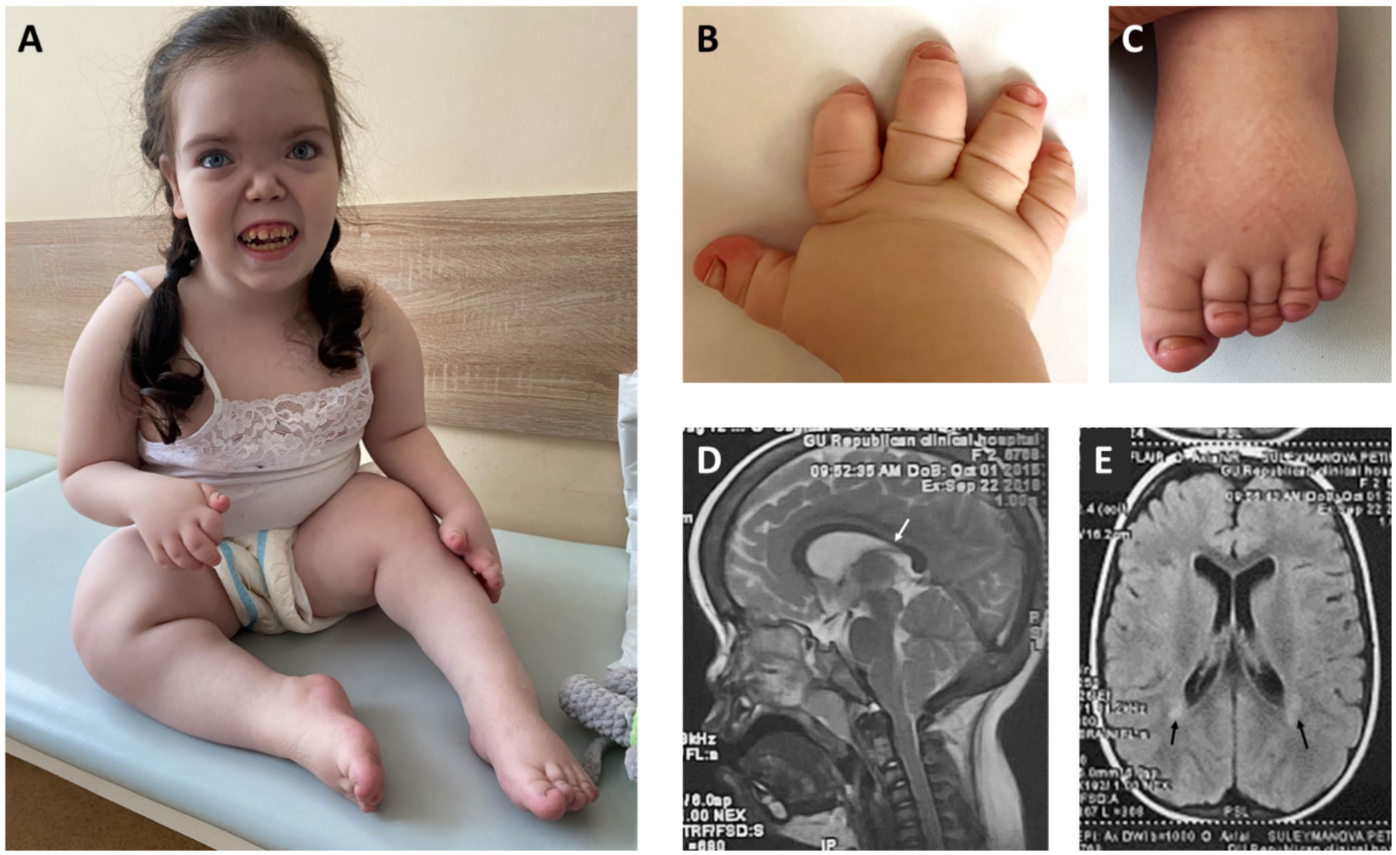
(A) - Facial features: broad forehead with prominent frontal bossing, hypertelorism, low nasal bridge, midface hypoplasia, long smooth philtrum, tented upper lip, protruding upper incisors, enamel hypoplasia, open mouth. Obesity, growth retardation with micromelia, knee joint contractures. (B,C) – Severe brachydactyly with nail hypoplasia. (D) – Midsagittal view of brain MRI – thinning of the isthmus of corpus callosum (white arrow). (E) – Axial view of brain MRI – periventricular leukomalacia adjacent to the external angles of the lateral ventricles (black arrows).
